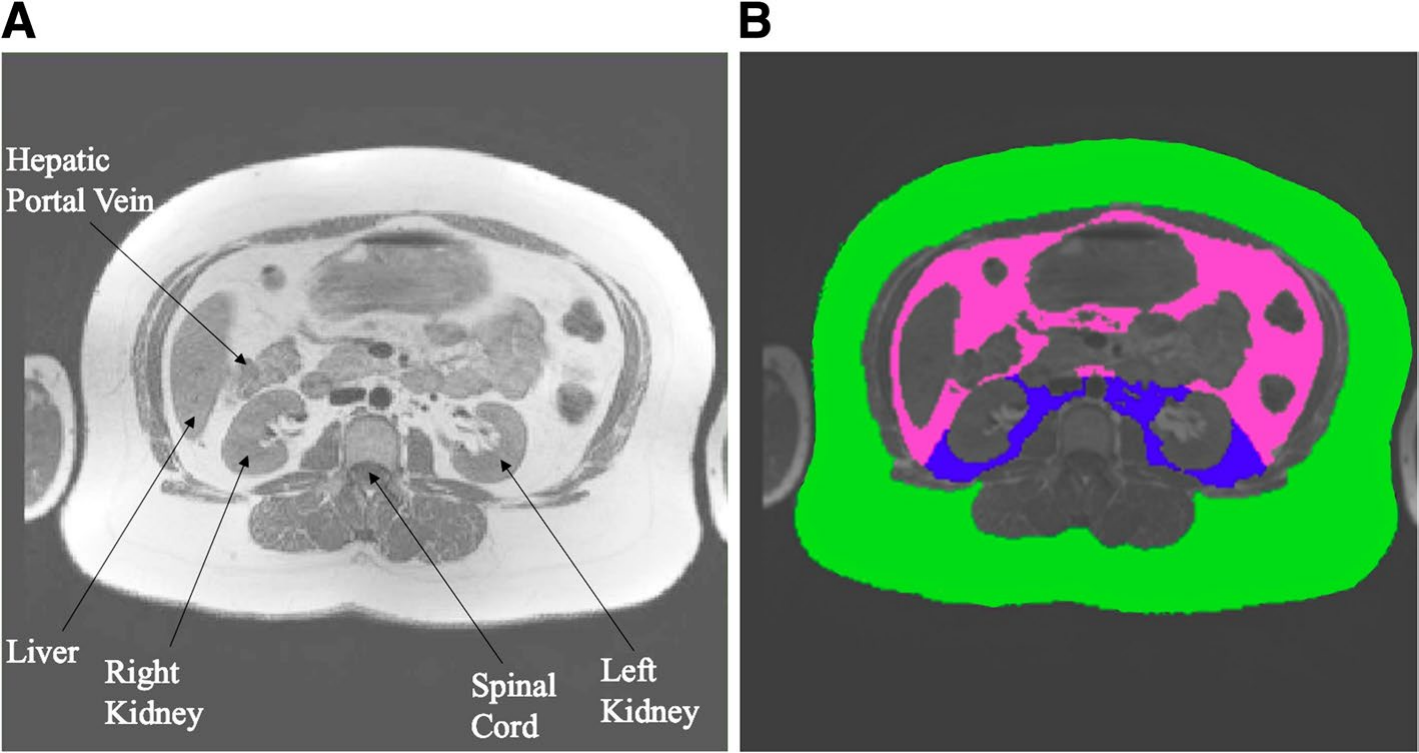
A Abdominal MRIs at the second lumbar vertebra were obtained. The hepatic portal vein, liver, kidneys, and spinal cord were visualized in the abdominal MRI. B From the image in Fig. 1A, color-coding was used to denote adipose tissue depots. The green represents subcutaneous adipose tissue, while the pink and blue represent intraperitoneal and retroperitoneal adipose tissue, respectively. Intra-abdominal adipose tissue was the combination of intraperitoneal (pink) and retroperitoneal (blue)
